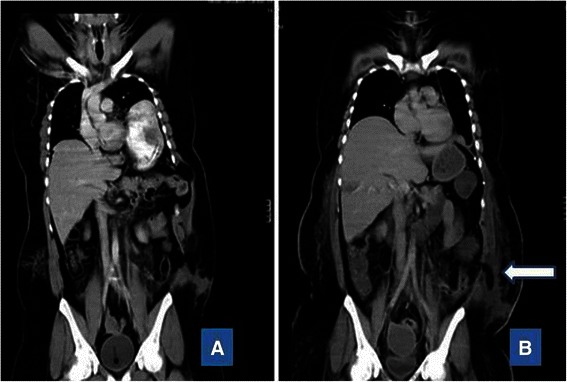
a Preoperative computed tomographic scan showing herniation of the patient’s stomach into the left thorax and of the small bowels into an abdominal wall defect. b Computed tomographic scan of the patient’s abdomen obtained at 2 weeks after the accident showing fat stranding and presence of air locules suggestive of infection of abdominal wall collection (*arrow*)
